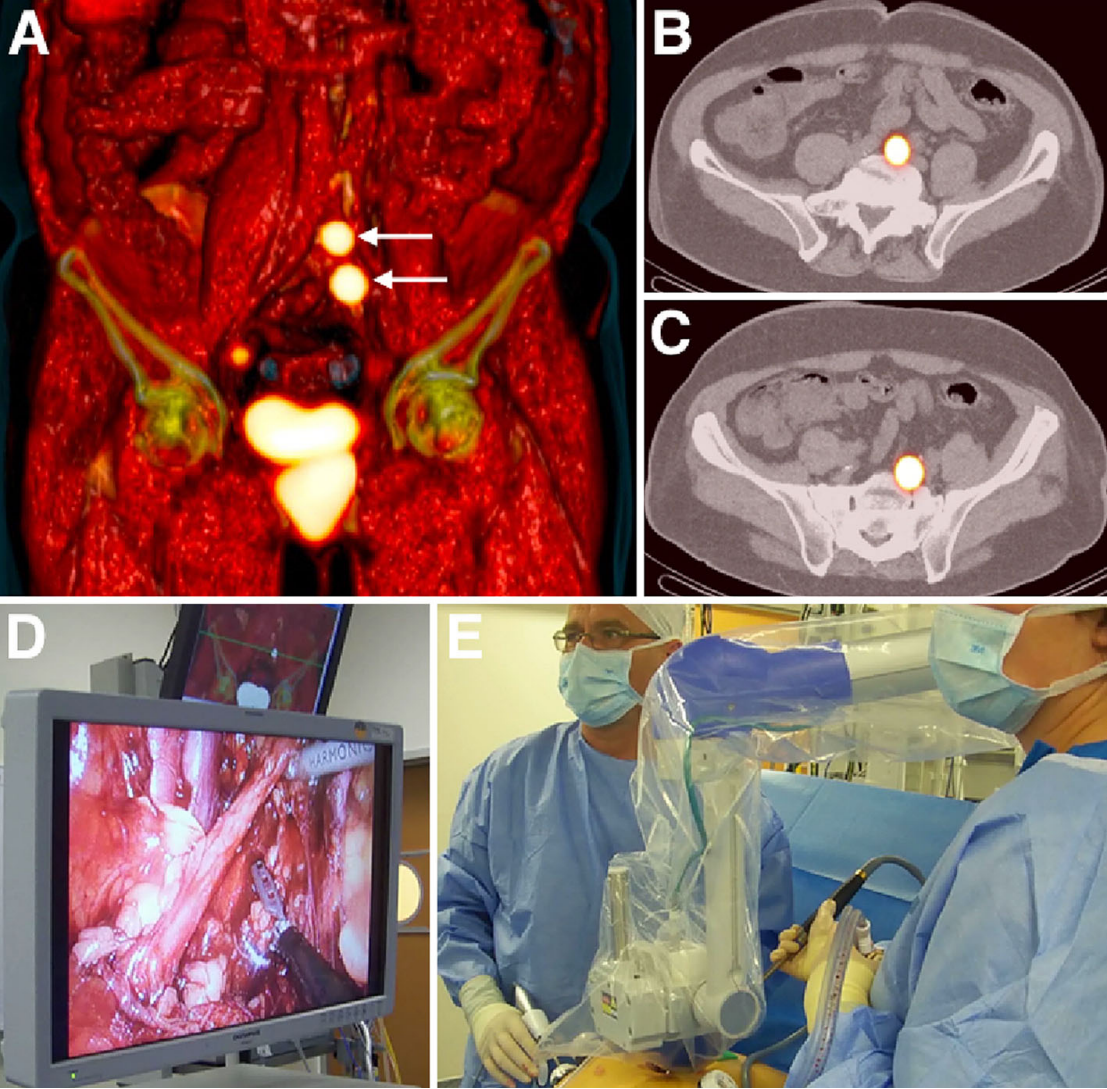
Sentinel node localisation in prostate cancer after administration of ^99m^Tc-nanocolloid. Coronal volume rendering (a) and transversal (b, c) SPECT/CT showing two sentinel nodes along the left common iliac artery. Based on this anatomical information both nodes are subsequently removed (d) laparoscopically, guided by a portable gamma camera (e)
